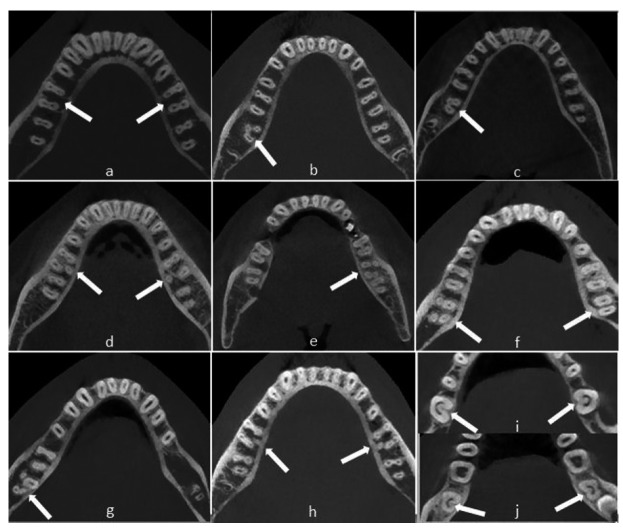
Cone-beam computed tomography images demonstrate: (a) Cases of mandibular bilateral first molars with two roots and two canals in axial section. (b) Case of mandibular right second molar with three root and mesiolingual root. Note that distal root fused with buccal part of mesial root (c) Case of mandibular right second molar with two roots. Note that distal root fused with buccal part of mesial root. (d) Distolingual roots can be seen concurrently in the first molars bilaterally. (e) Cases of mandibular left second molar with mesiobuccal 2-distobuccal 2 roots. (f) Cases of mandibular right second molar with four roots and left second molar with two roots- two canals. (g) Cases of mandibular right second molar with mesiobuccal 2-distolingual 2 roots. (h) Cases of bilateral mandibular first molars with five-canal and three roots. (i,j) Cases of bilateral mandibular first and second molars with C-shaped canals.
